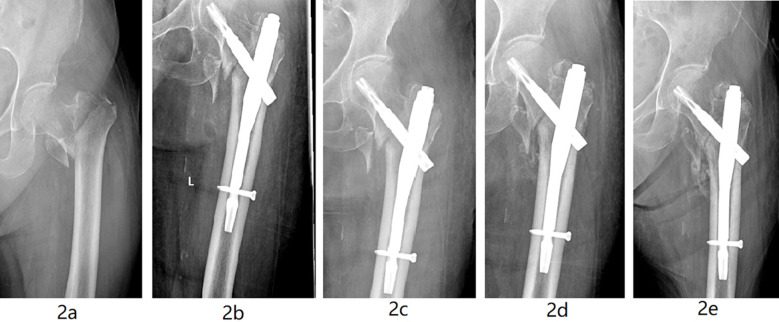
PFNA internal fixation in a 68-year-old female patient with a left intertrochanteric fracture. 2a: X-ray image showing intertrochanteric fracture of the femur after the injury. 2b: Postoperative X-ray showing appropriate fracture reduction. 2c: X-ray image showing appropriate internal fixation position evolution one month after the operation. 2d: X-ray image showing appropriate bone union of fracture six months after the operation. 2e: X-ray image showing appropriate fracture healing 12 months after the operation.
